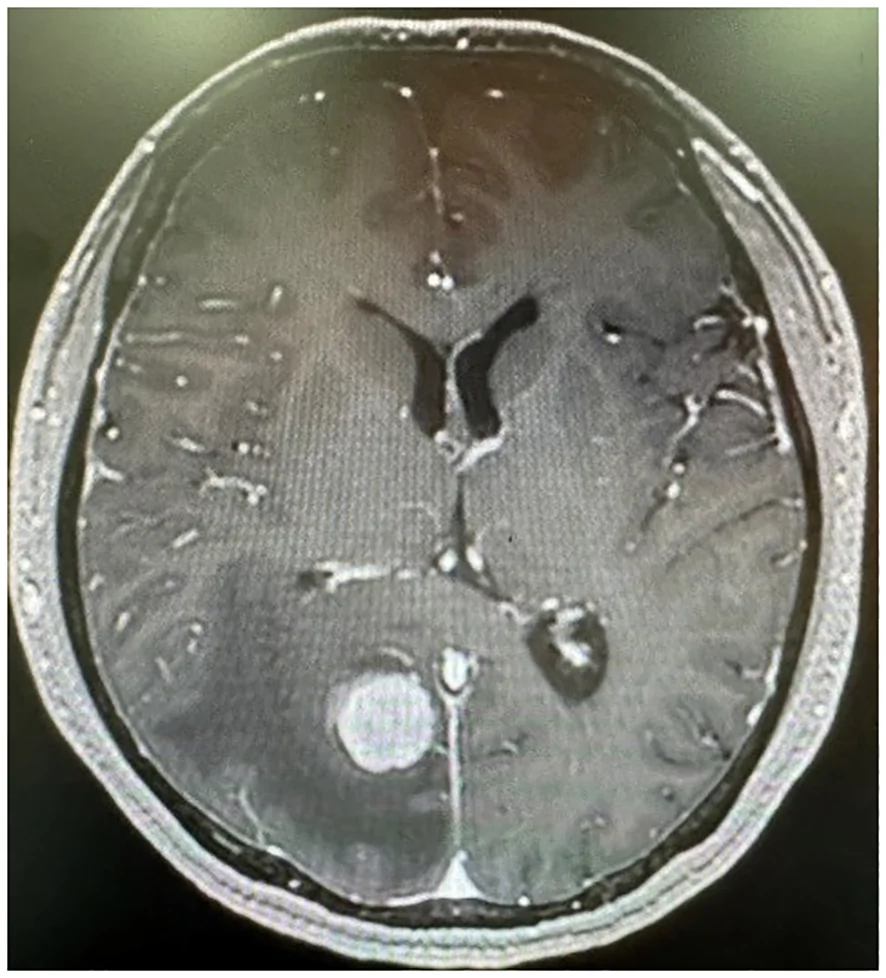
Brain MRI from March 2024 (THK:1.6mm). A new metastatic lesion, measuring approximately 2.3 × 2.0 cm, was identified in the occipital lobe, demonstrating moderate heterogeneous enhancement and surrounding edema.
